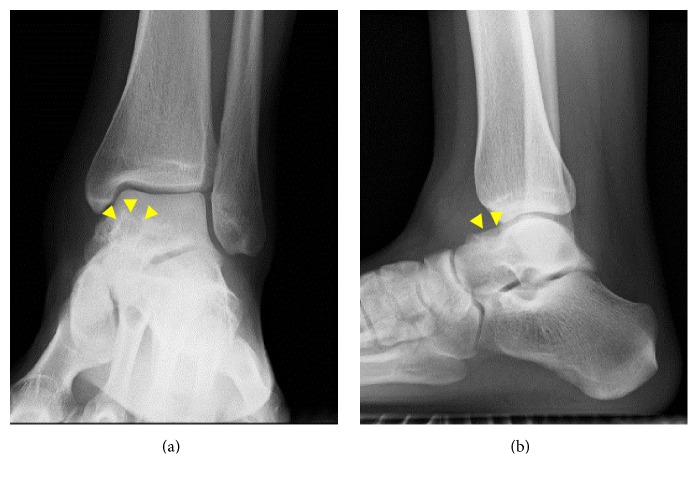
Anteroposterior (a) and lateral (b) plain radiographs of the left ankle showing a radiolucent lesion with marginal sclerosis in the talar neck (arrow-heads).
